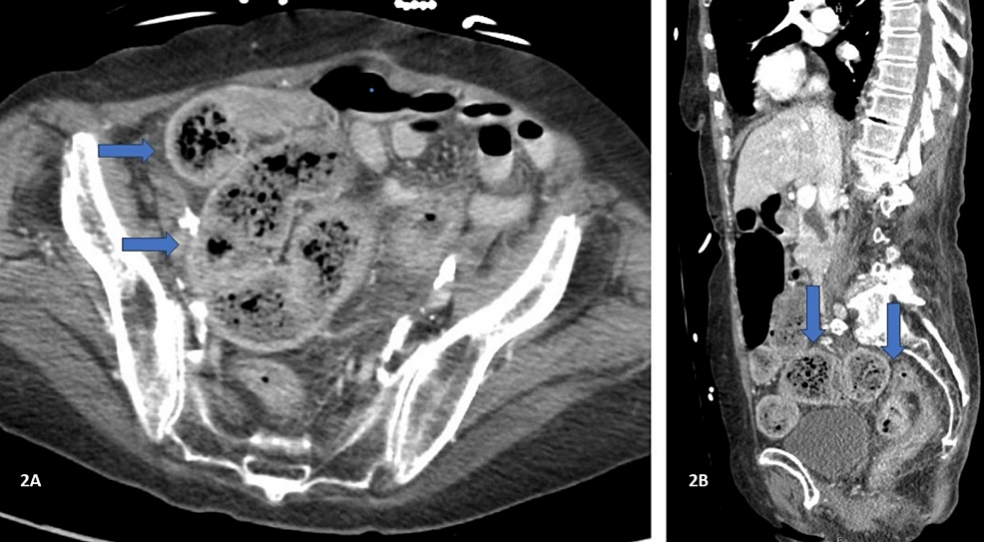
CT with contrast of the abdomen and pelvis - image 1 Impression: a large amount of stool within the colon, which is compatible with constipation. There is colonic thickening and induration involving the rectum and sigmoid colon; there is marked dilatation of the cecum. Compatible with colitis in the proper clinical setting CT: computed tomography
